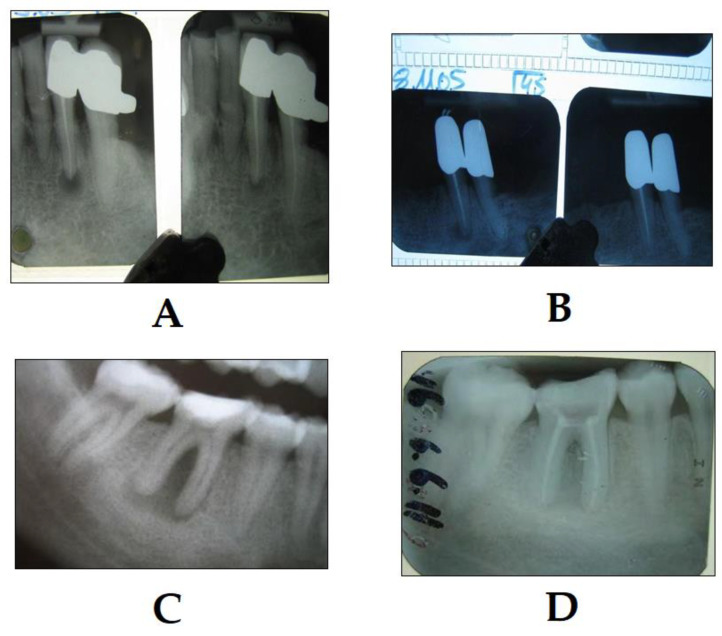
Success of PDT in the treatment of chronic periapical periodontitis (photo courtesy of R. Szabó DMD, J. Arentz DMD and T. Nave DMD). (A) The X-ray on the left shows the condition immediately after PDT, and the X-ray on the right shows the condition 4 months later; note the significant decrease in the size of the periapical lesion; (B) the X-ray on the left shows the condition immediately after PDT, and the X-ray on the right shows the condition 12 months later; note the significant decrease in the size of the periapical lesion. The same case is depicted in a lower molar tooth with chronic periodontitis, where (C) shows the condition immediately after the endodontic treatment and PDT, and (D) shows the condition after 3 months after the endodontic treatment, PDT therapy and root canal obturation; note the significant decrease in the size of the periapical lesion.
